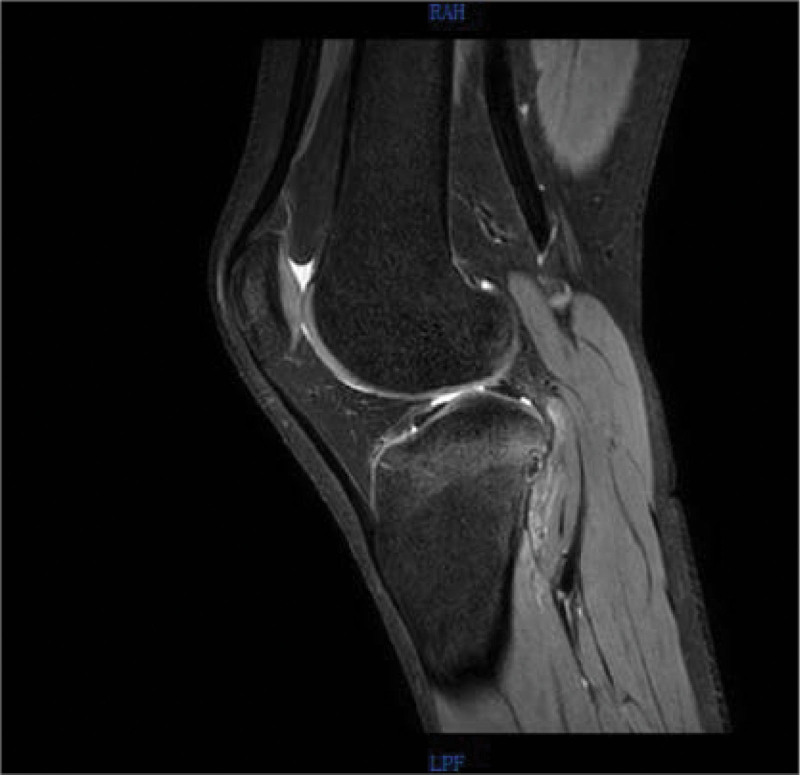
Preoperative MRI in mid-sagittal plane. MRI = magnetic resonance imaging.
